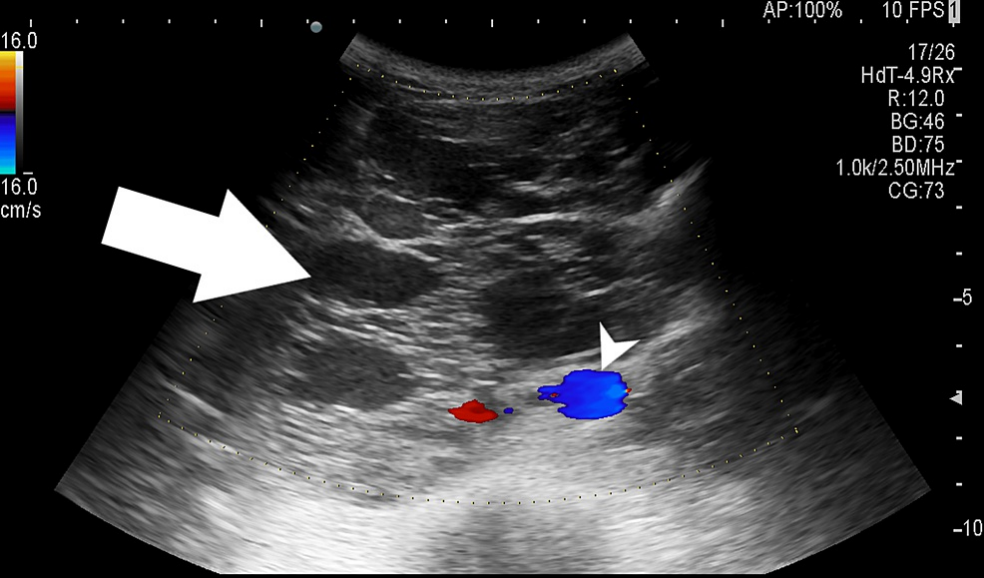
Ultrasound gray-scale image of the abdomen showing a large ill-defined multicystic mass in the retroperitoneum (arrow) with no internal vascularity on color flow. Arrowhead showing abdominal aorta being encased
